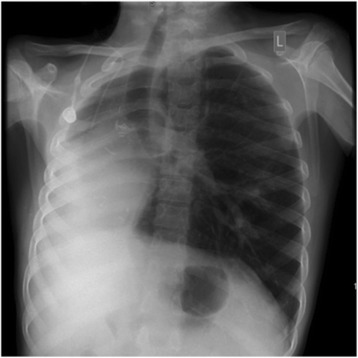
Chest radiograph around 6 months post-pneumonectomy
